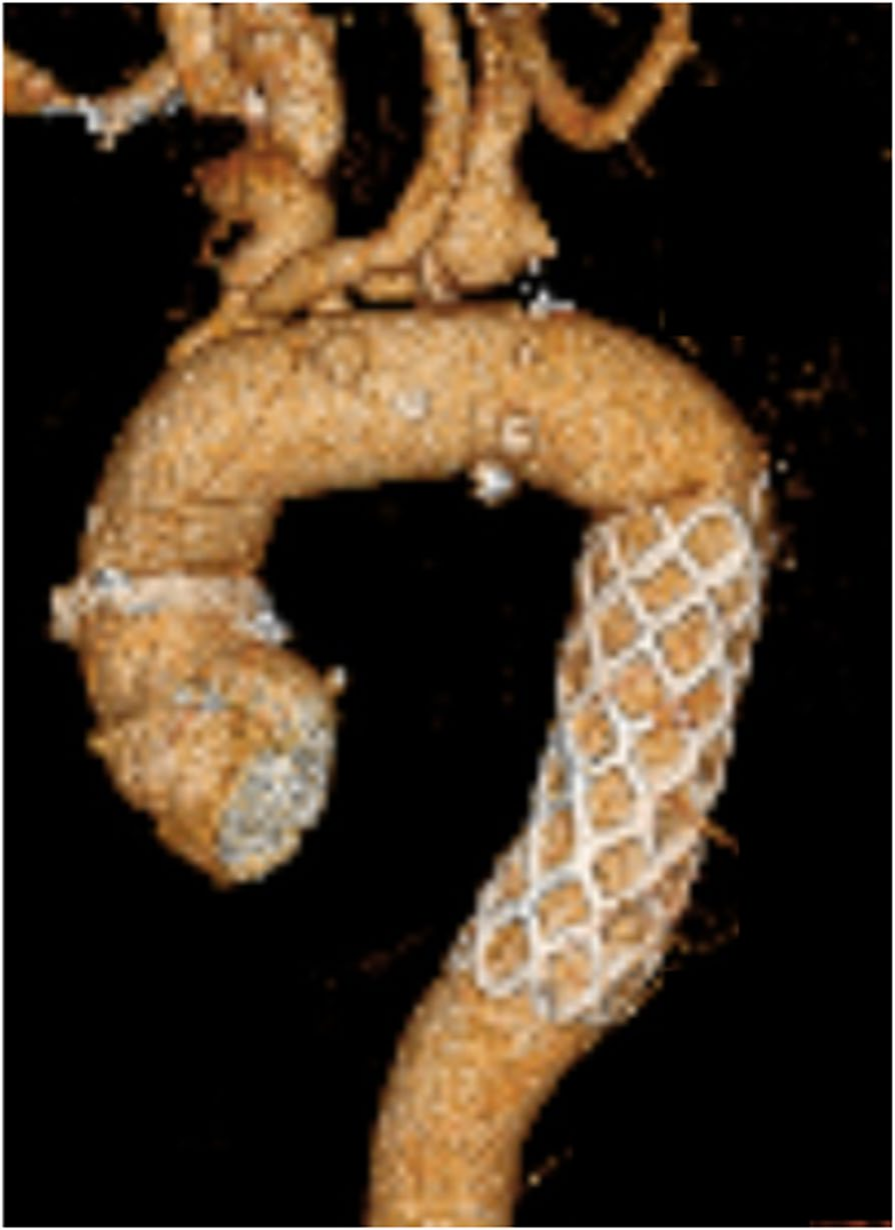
Three-dimensional computed tomography. Postoperative view. The coarcted aorta was resected, and the frozen elephant trunk was inserted into the descending aorta
